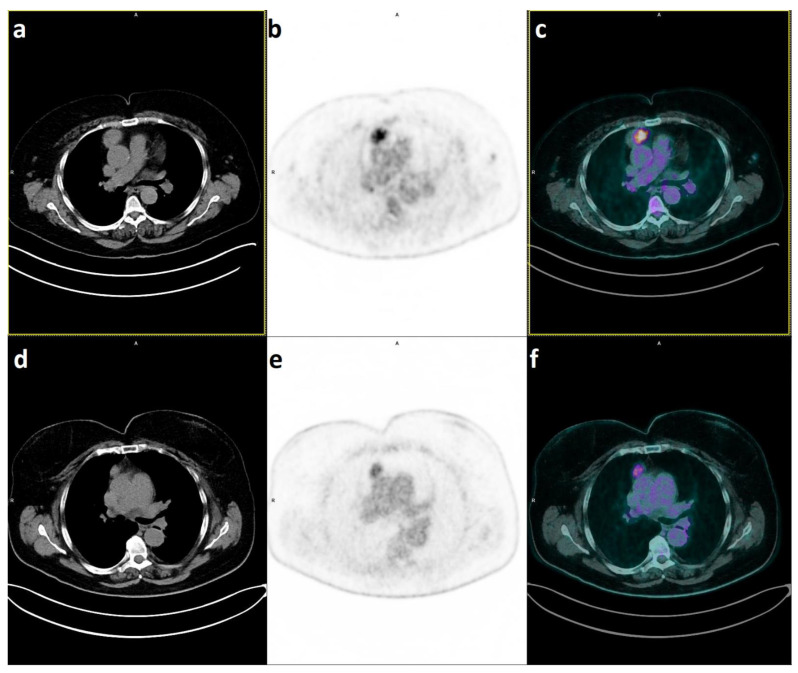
PET CT scan showing the absence of mild uptake in a case of Type AB-Thymoma (a–c) [18] compared with intense uptake in a Type B3-Thymoma (d–f) [36].
